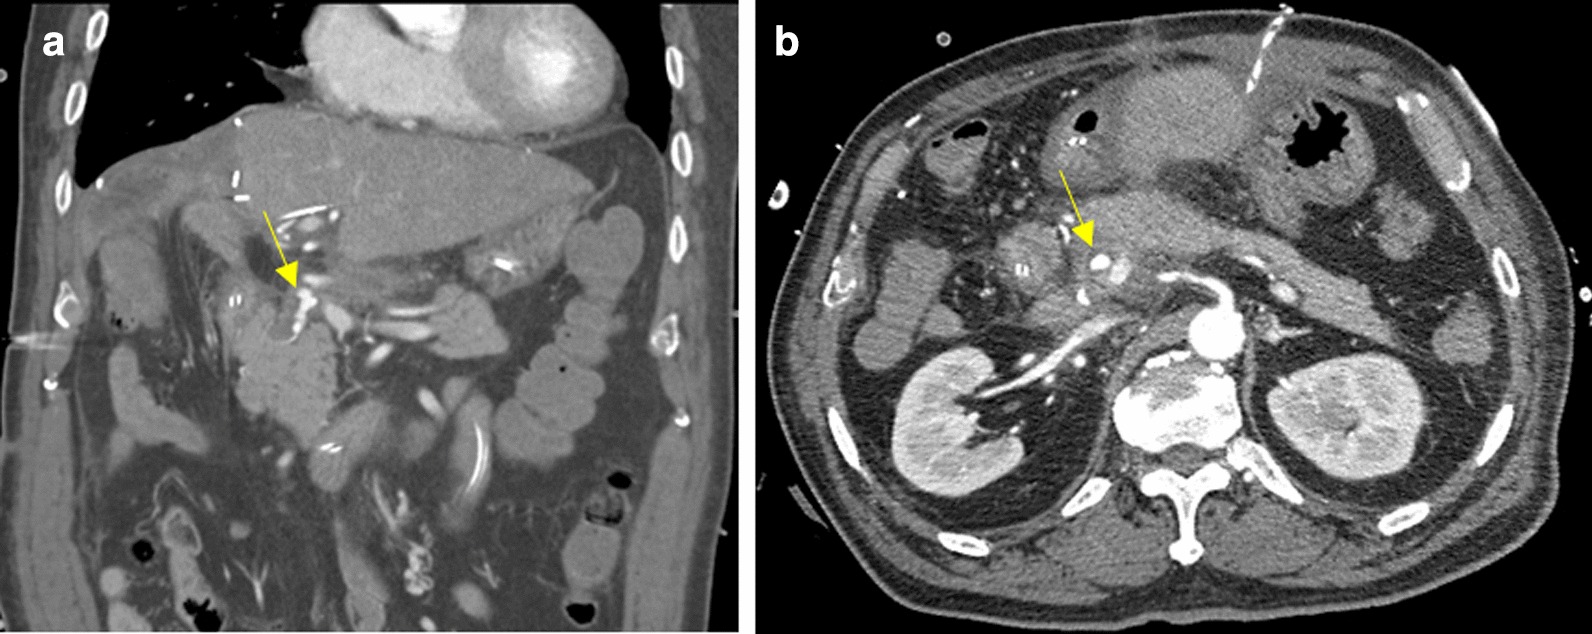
Arrows show the pseudoaneurysm of the GDA in the residual intra-pancreatic bile duct in Coronal (a) and Axial (b) contrast-enhanced abdominal CT images
